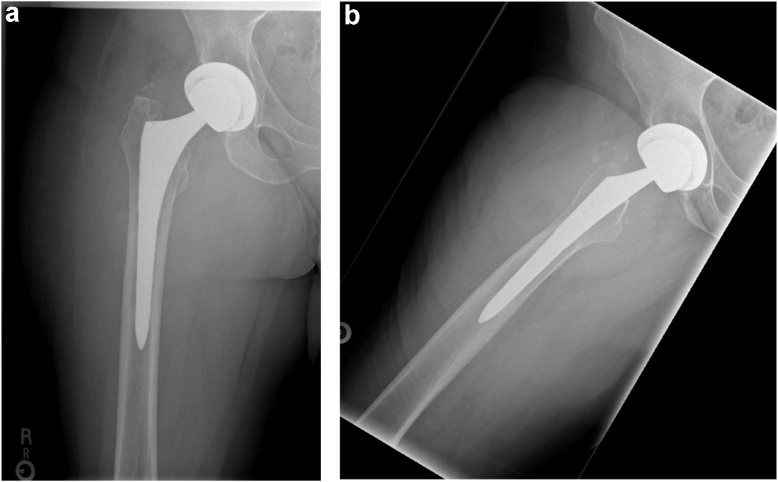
(a) Anterior-posterior (AP) and (b) frog leg lateral radiographs of the right hip 1-month status postrevision to ceramic-on-polyethylene bearing.
